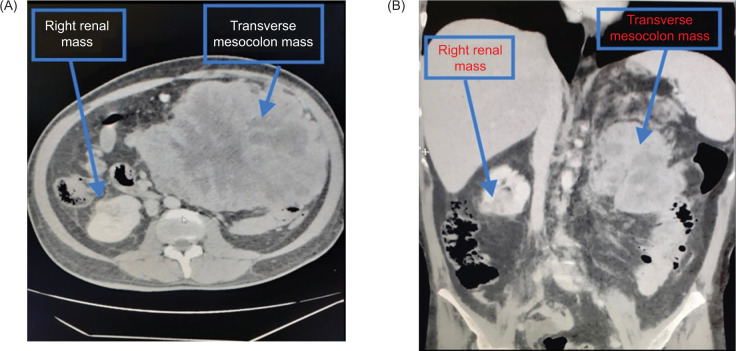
(A–B) Contrast-enhanced CT showing heterogeneously enhancing mass adjacent to the transverse colon and right lower pole kidney mass.
